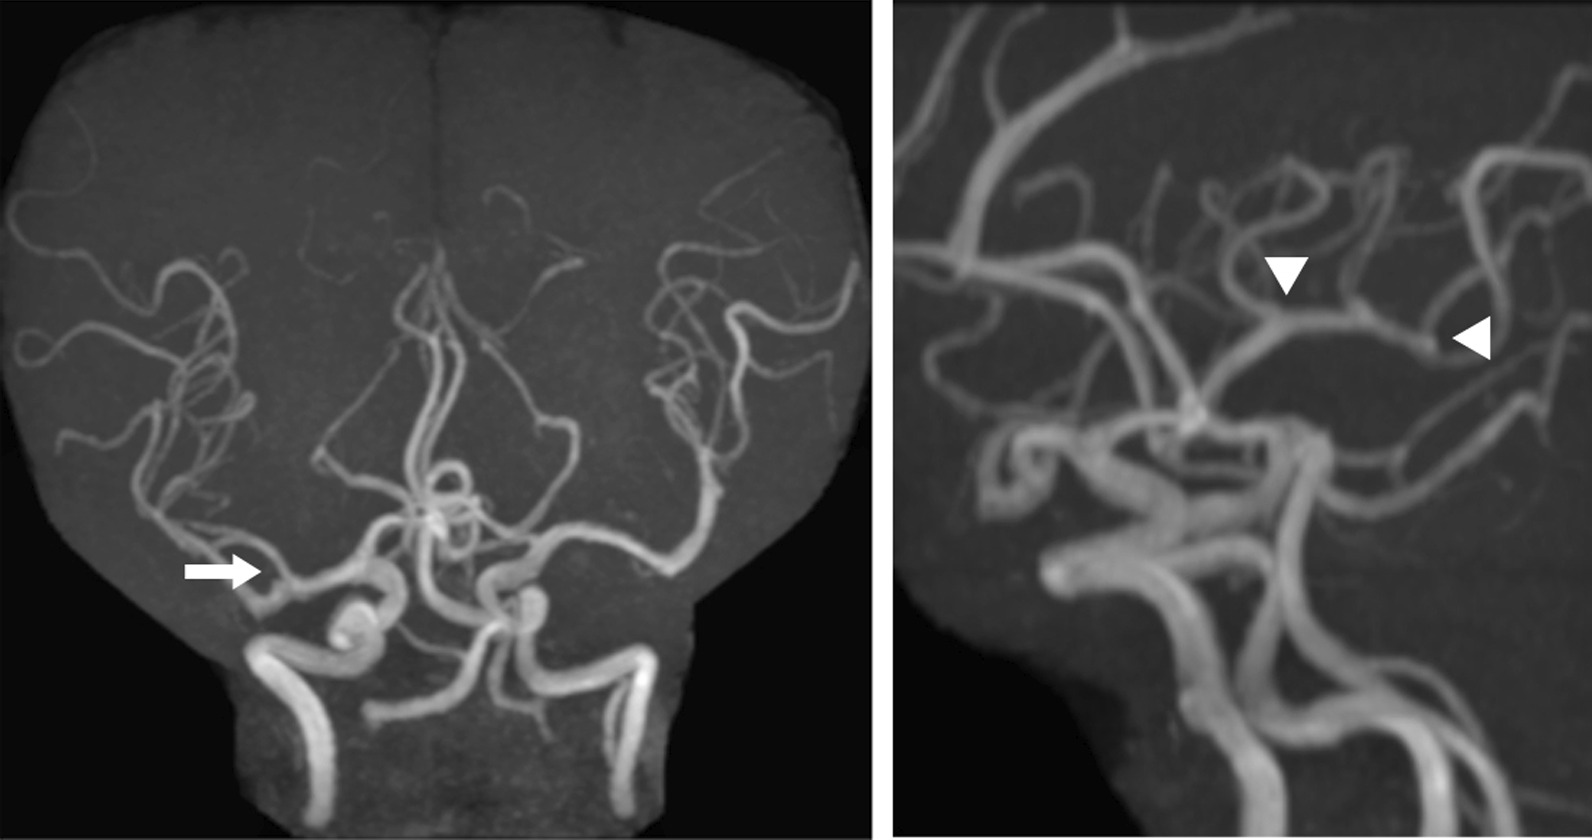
Magnetic resonance imaging (MRI) in the previous year. Left: because a small intracranial aneurysm (arrow) was found in the right middle cerebral artery 2 years ago, she underwent annual MRI follow-up. Right: in the previous year, there had been no aneurysms in the left middle cerebral artery where the aneurysms were (arrow head)
